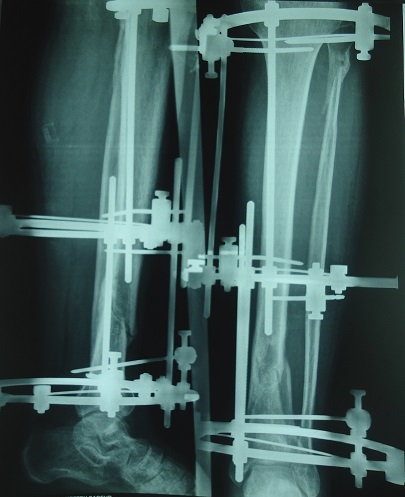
Immediately postoperative X-ray shows good reduction of the pilon fracture
